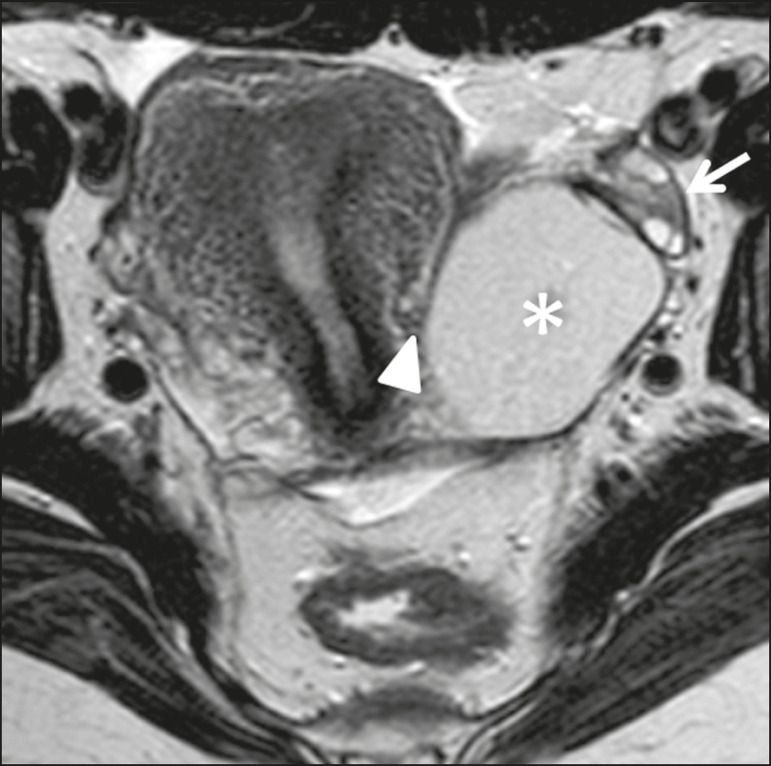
Serous cystadenoma of the broad ligament in a 19-year-old woman. Axial T2-weighted imaging showing a unilocular cystic tumor (asterisk) clearly demarked from the left ovary (arrow) and the uterus (triangle).
